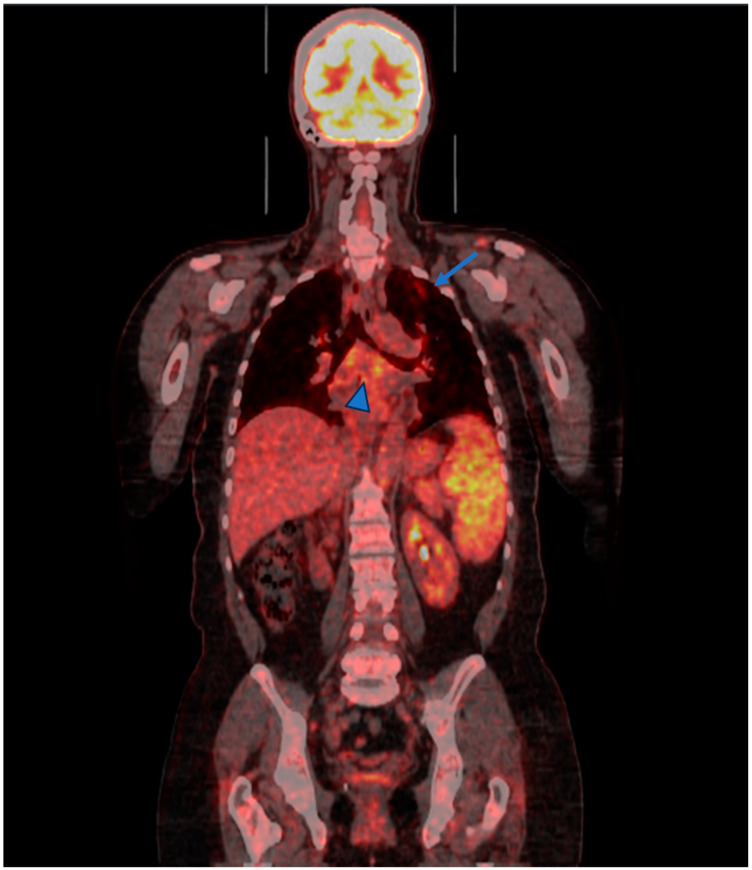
65 years old woman affected by sarcoidosis. Disease activity is prevalent in the left upper lobe (arrow) sub-carinal lymph node (arrow head) and spleen (mild enlargement, high and dishomogeneous FDG uptake).
